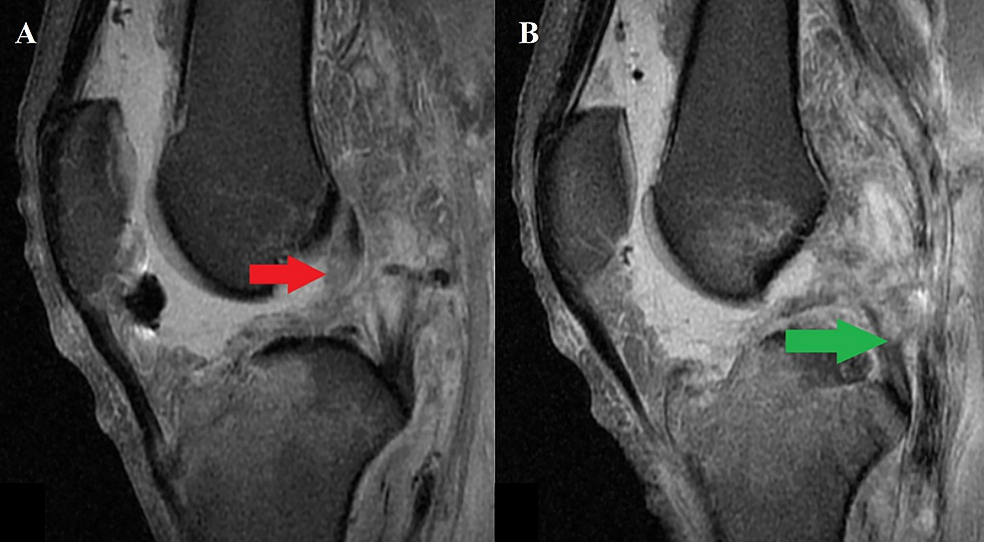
Preoperative MRI of the left knee. Left knee preoperative MRI shows ACL (red arrow) and PCL (green arrow) disruption, large effusion, and significant soft tissue edema. ACL: anterior cruciate ligament; PCL: posterior cruciate ligament; MRI: magnetic resonance imaging
